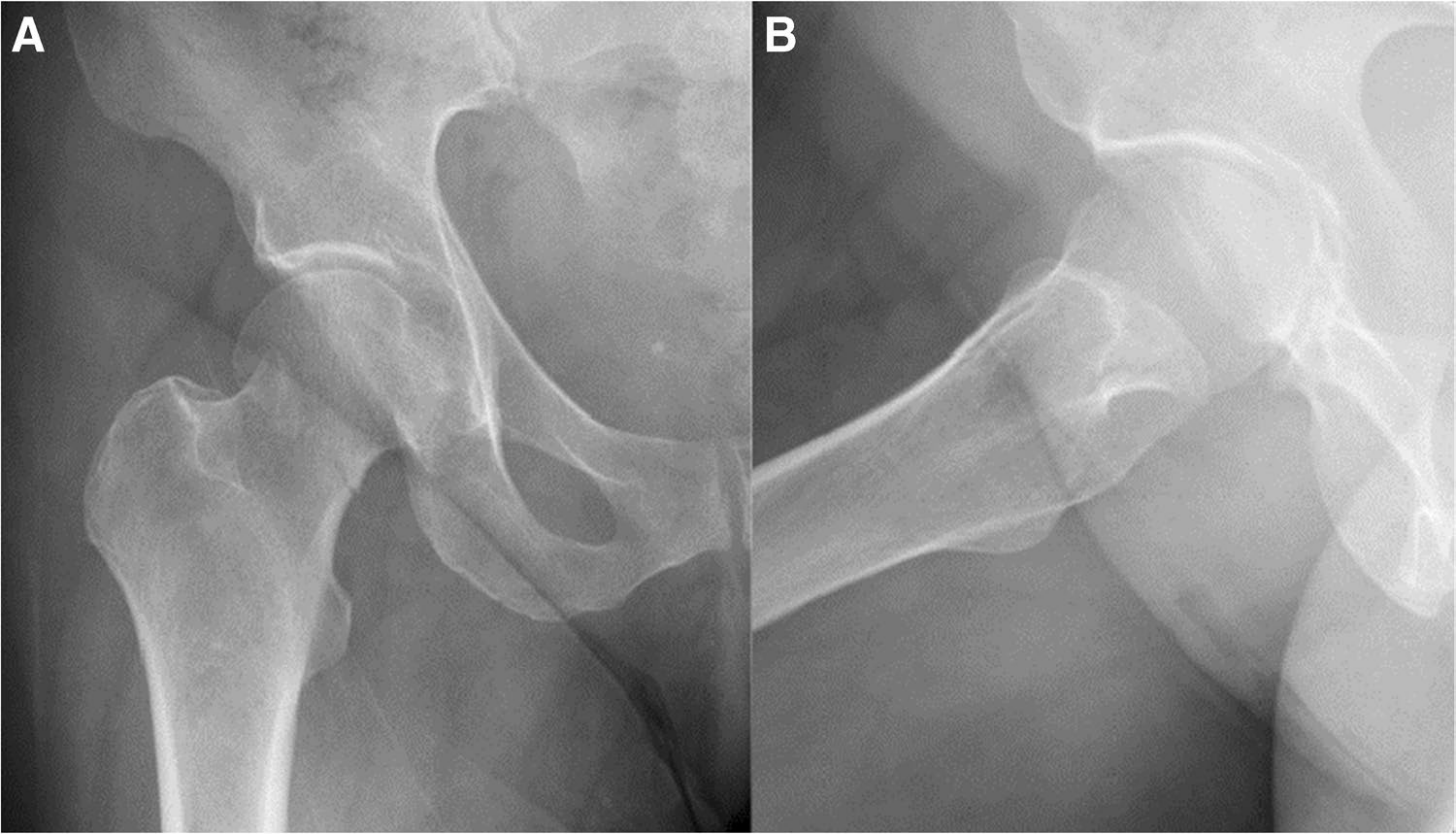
A, B AP and lateral radiographs showing right intracapsular femur fracture of a 71-year-old female. An isolated injury to the right hip after falling from own height when getting out of bed. Past medical history consists of chronic lymphocytic leukaemia, walks unaided
